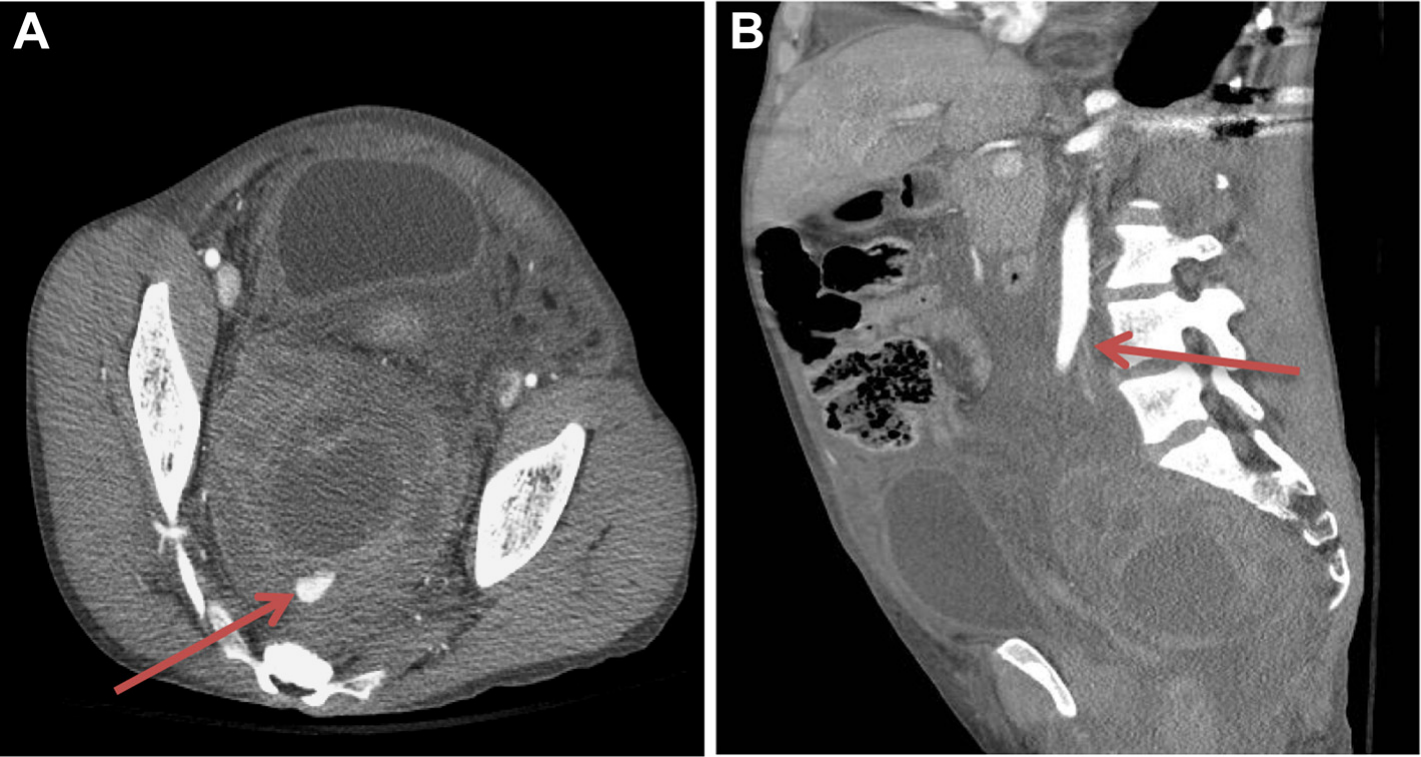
a Axial contrast-enhanced computed tomography scan showing a large heterogeneous retroperitoneal/pre-sacral hematoma with active extravasation (arrow). b Sagittal contrast-enhanced computed tomography scan showing the origin of the median sacral artery from the distal abdominal aorta (arrow)
